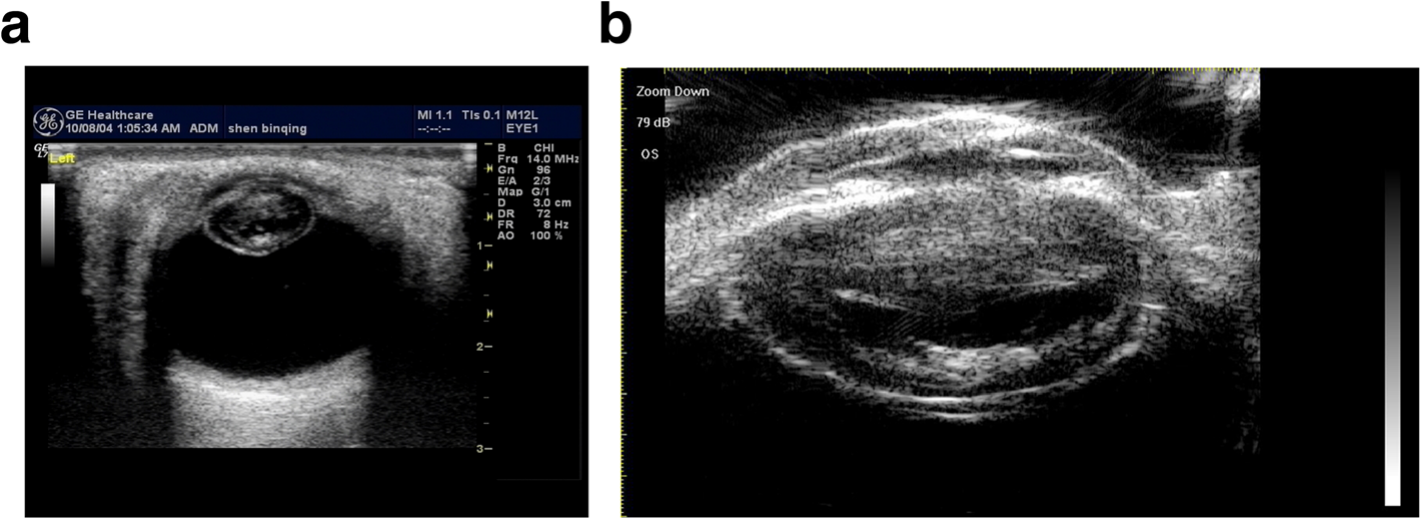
a The true negative image taken with 14-MHz + THI. The PC is smooth and round with a continuous echo. b: The true negative LFUBM image. The ultrasonic performance is similar to that shown in a
